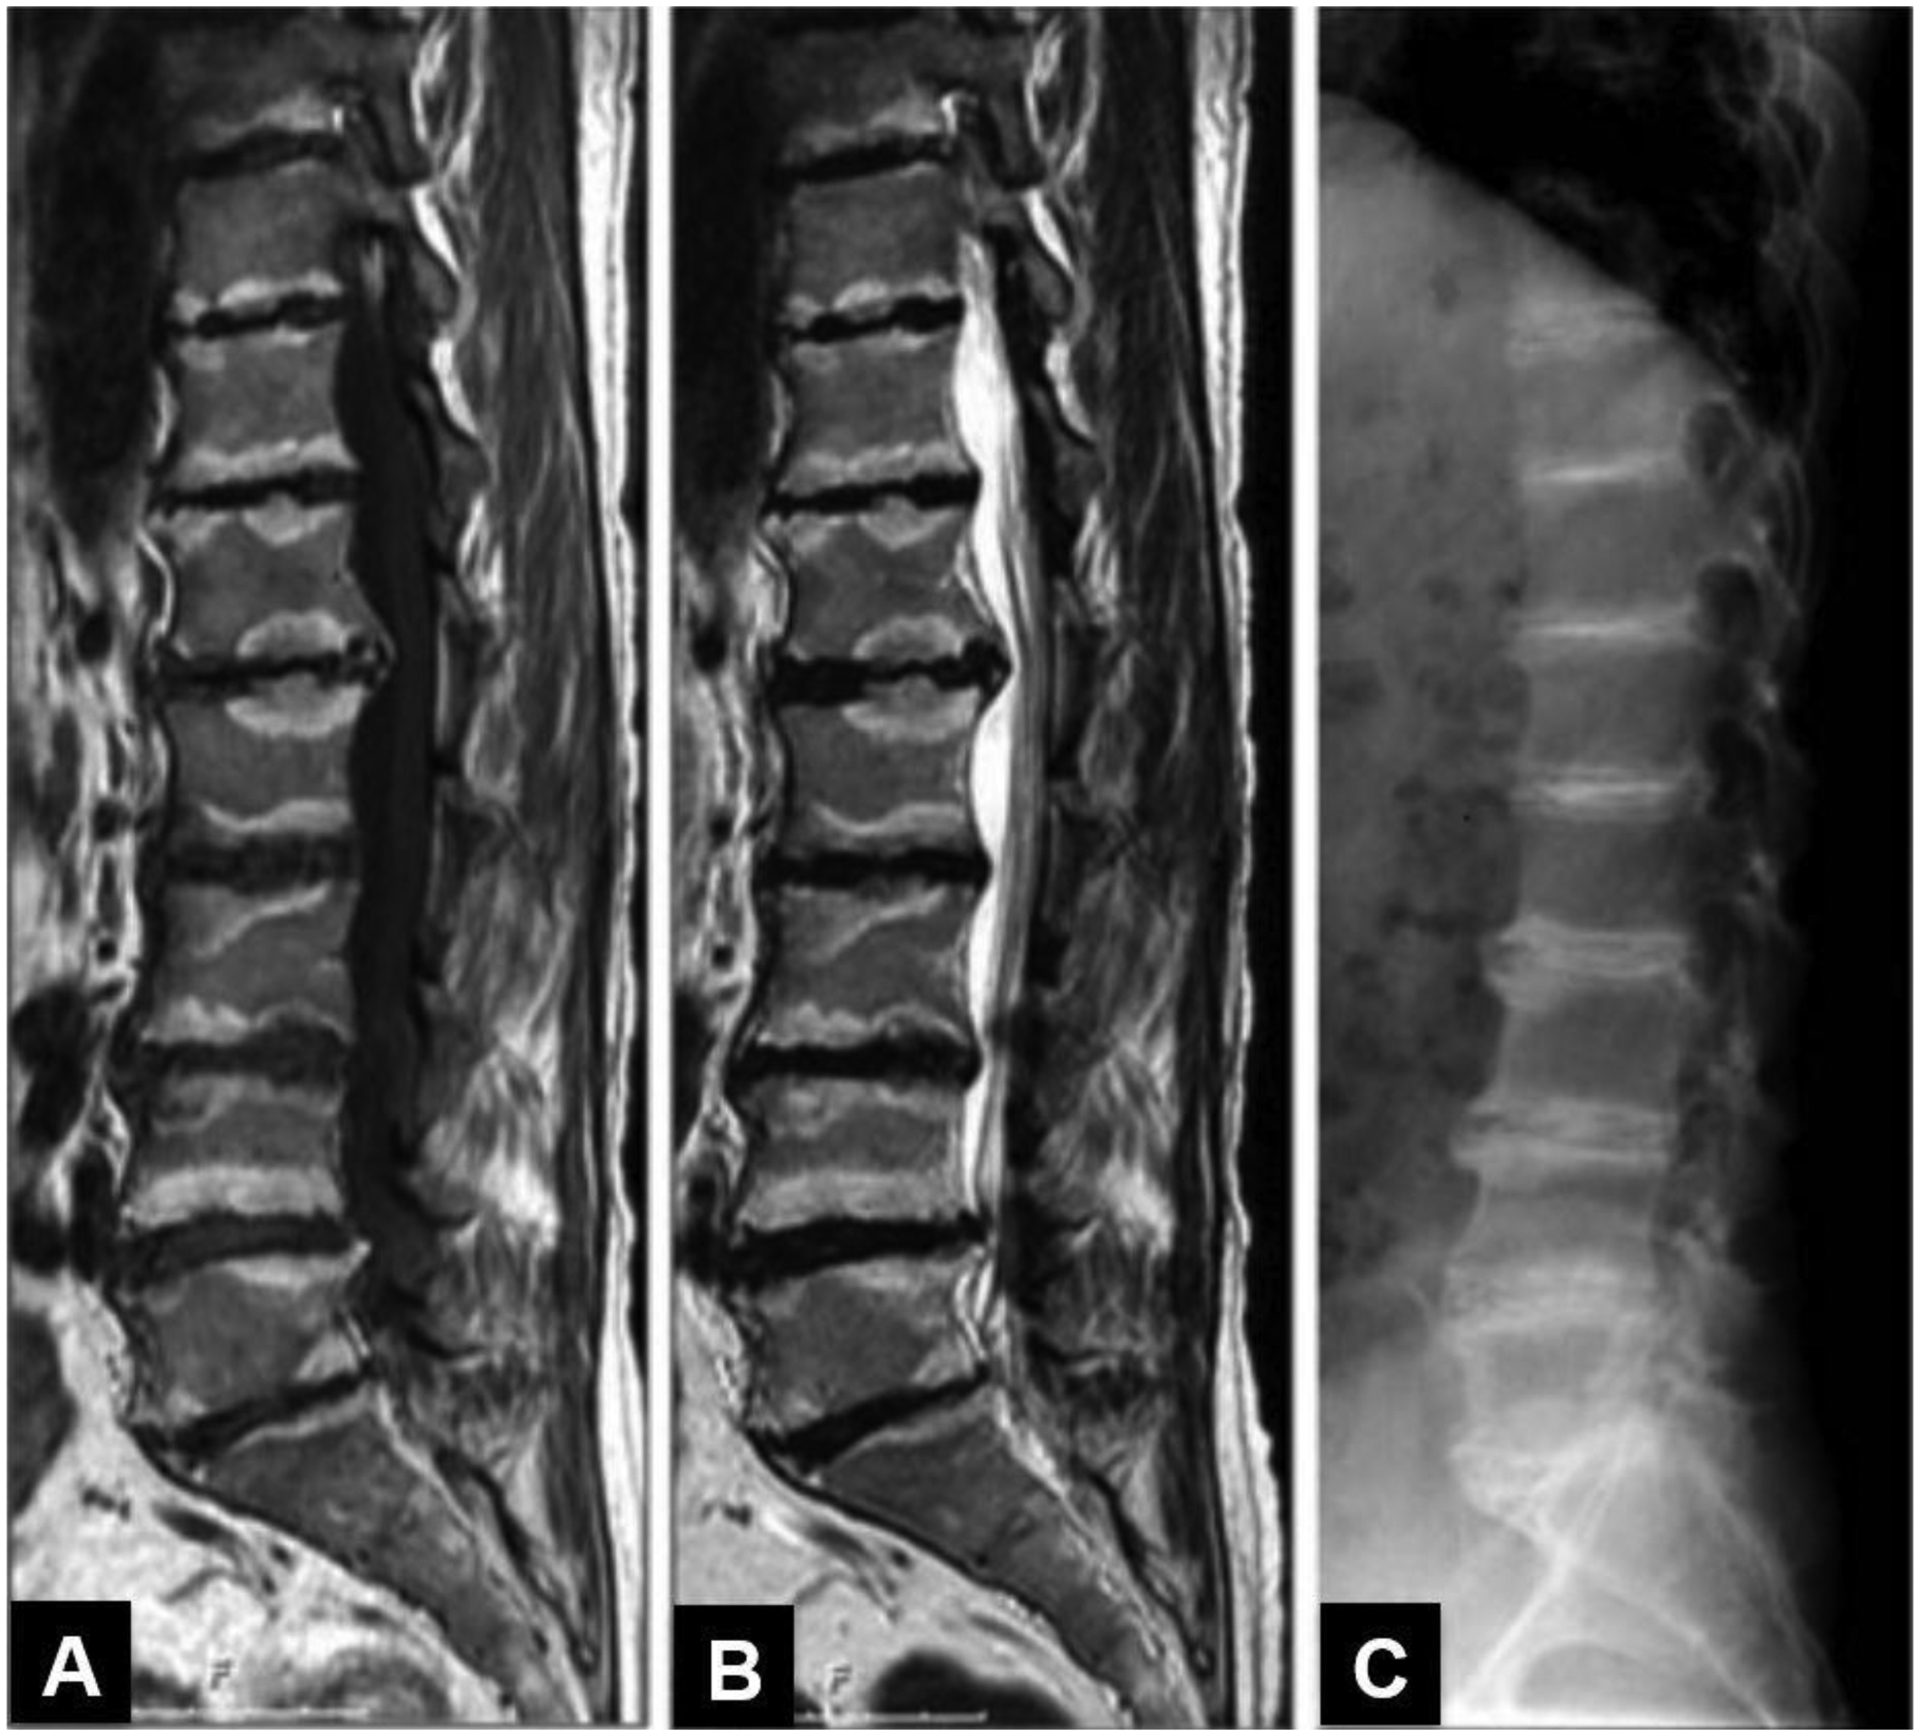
50- year- old man with ochronosis. Magnetic resonance imaging (MRI) (a and b) and radiography (c) of the lumbar spine, with classic changes of ochronosis. Reproduced with permission [15].
